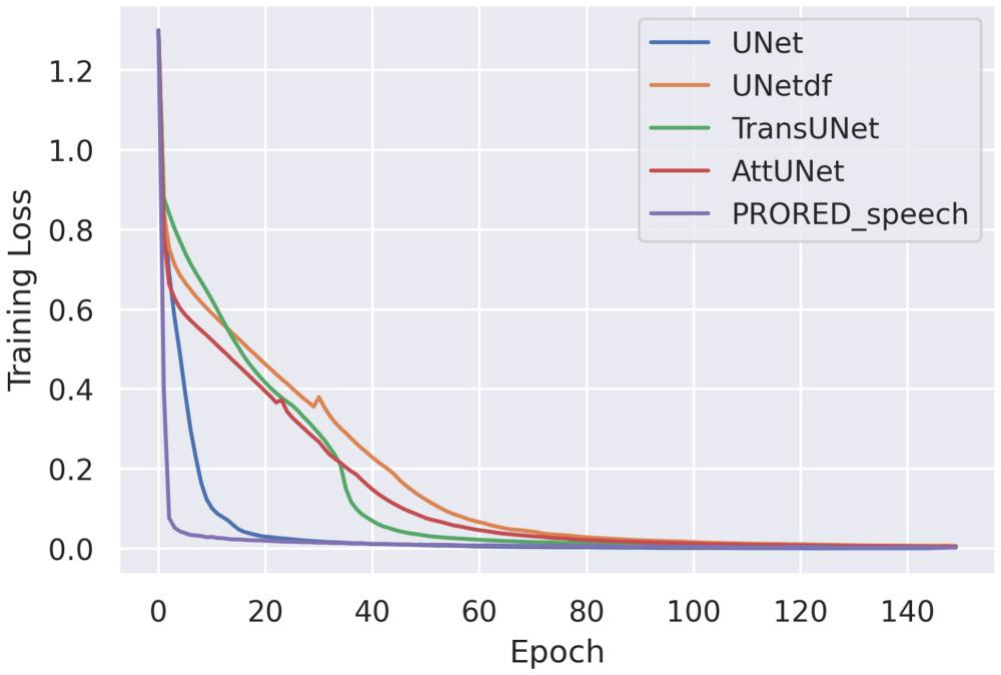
Training loss plots for the baseline and proposed models from one of the folds on speech MRI data.
